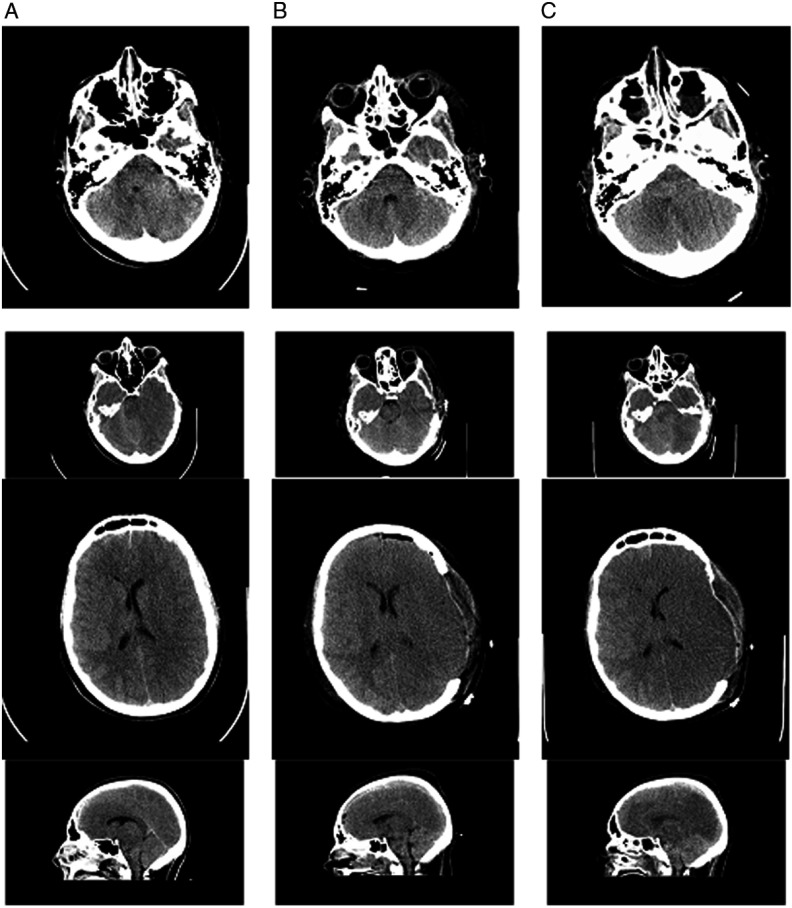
Additional Images of non-contrast CT head imaging 2 hours after the patient’s injury (column A), 2 days post-injury (column B), and 3 days post-injury (column C). There is initial generalized cerebral edema with early midline shift and a small left-sided SDH. The progression of cerebral edema and loss of gray–white matter differentiation, despite surgical and medical intervention including craniectomy, can be seen.
